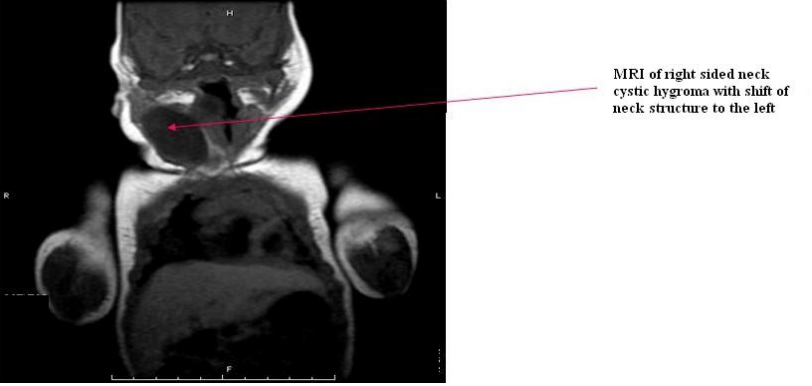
Initial magnetic resonance imaging of the neck showing right sided cystic hygroma, pushing the neck structures to the left.
